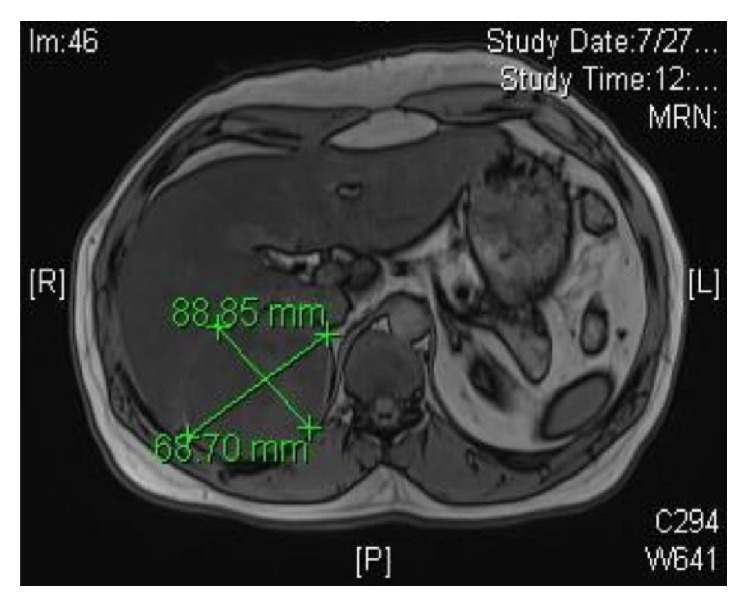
MRI (R) adrenal mass 88 × 68 mm.
